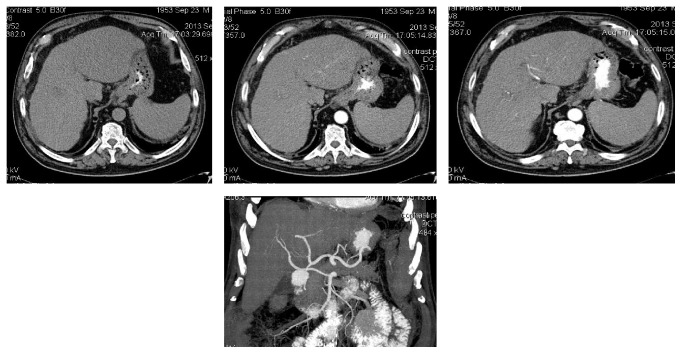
Preprocedure CT (native and arterial phase). Note: superior mesenteric artery type right hepatic artery; left lobe mass main feeder is left hepatic artery.
